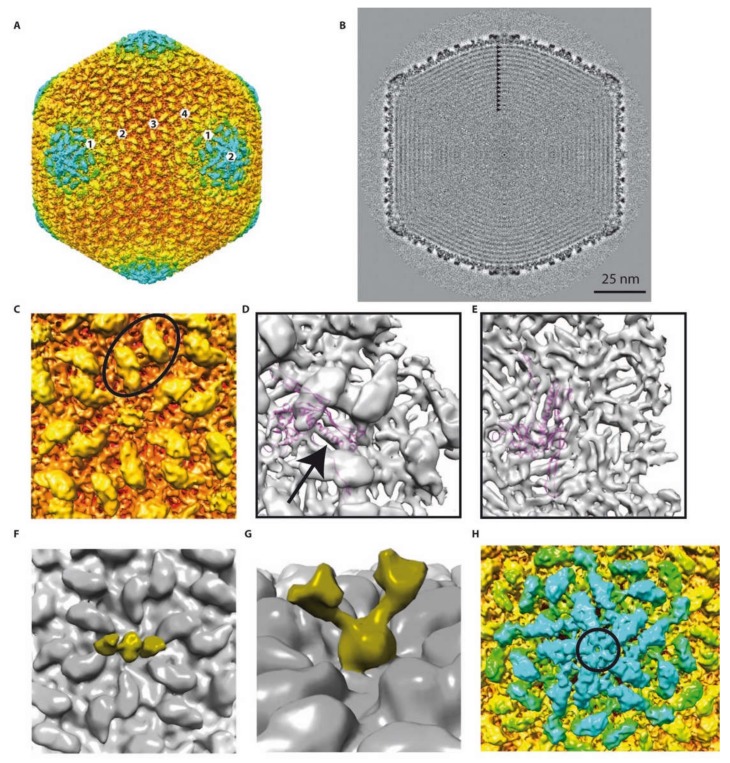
Detailed 3D reconstruction of the phAPEC6 capsid: (A) Isosurface representation of the phAPEC6 head 3D reconstruction at a 10-Å resolution. The particle is colored according to its diameter (from red to blue). (B) Central slice of the phAPEC6 head visualizing 15 concentric layers of DNA (black arrows). (C) Close-up view of one 6-fold axis. The major capsid protein (MCP) is colored in orange and the decoration protein in yellow. The black oval highlights a local 2-fold axis. (D) Fitting of the HK97 MCP X-ray structure into the phAPEC6 hexamer density seen from the outside of the particle (an arrow highlights the long alpha helix present in both HK97 and phAPEC6 MCP) and (E) seen from the inside of the particle. (F) Detail of the hexamer central protein along a 2-fold axis, displaying the fiber-anchoring protein. (G) Side view of the fiber-anchoring protein. (H) Close-up view of the 5-fold axis. The pentamer is colored in blue and highlighted by a black circle. The same protein as the one colored in yellow in part C is visible around the 5-fold axis.
